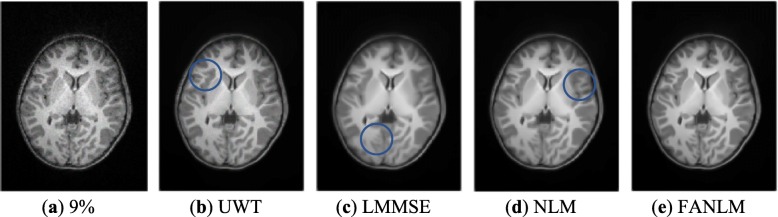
Experimental results of different methods. (a) corrupted MR image with 9% Rician noise; (b) UWT; (c) LMMSE; (d) NLM; (e) FANLM
